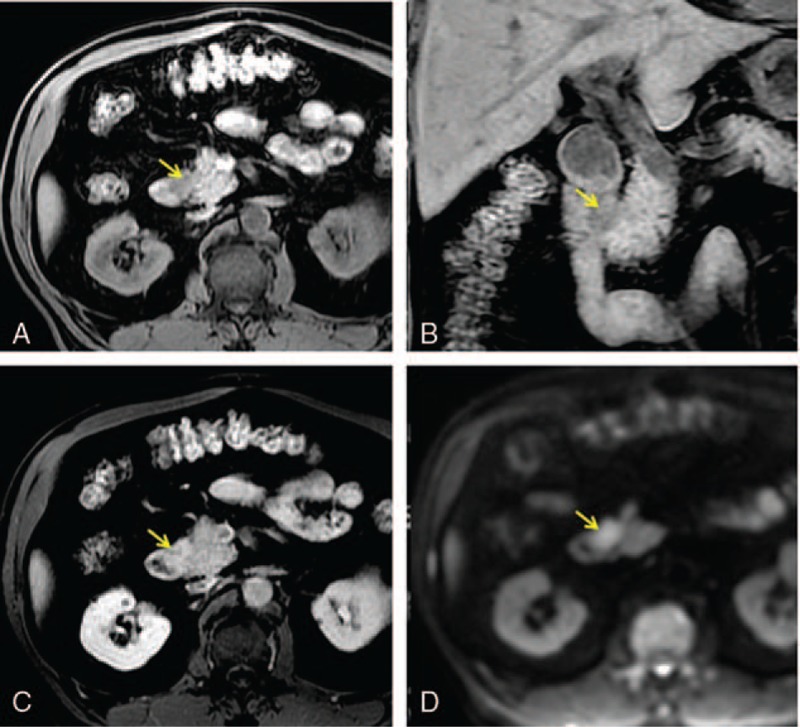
Axial (A) and coronal (B) T1-weighted fat-suppressed gradient-recalled echo MR image reveals a 2.0-cm hypointense lesion (arrow) in pancreatic groove. (C) The tumor becomes isointense in late arterial phase after contrast administration. (D) Diffusion-weighted MR image (b = 800 s/mm^2^) shows hyperintensity suggesting restricted diffusion.
